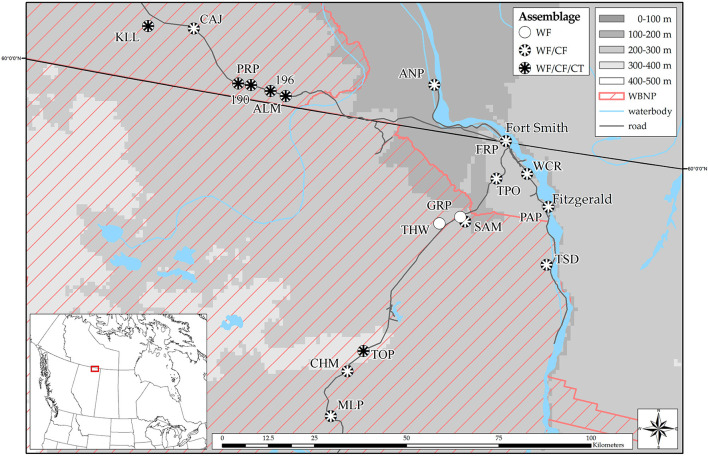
Amphibian communities sampled in Alberta and the Northwest Territories in 2016 and 2017 (WF, wood frog; CF, boreal chorus frog; CT, Canadian toad; WBNP, Wood Buffalo National Park). Map was created using ArcMap10.5 (Esri, Redlands, CA, USA).
